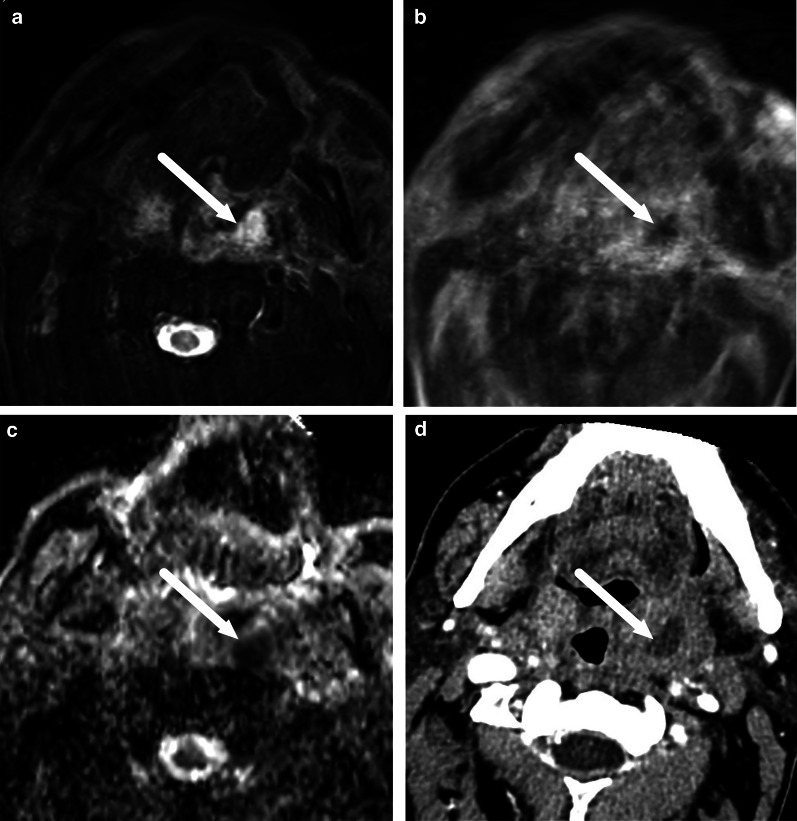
Excessive patient movement during scanning deteriorating image quality. Axial fat-suppressed T2-weighted (a), post-contrast T1-weighted (b), and ADC maps (c) demonstrate a left-sided PTA (arrows). The radiologist was left unsure about the true extent of the abscess in motion-degraded post-contrast images and recommended CT (d)
